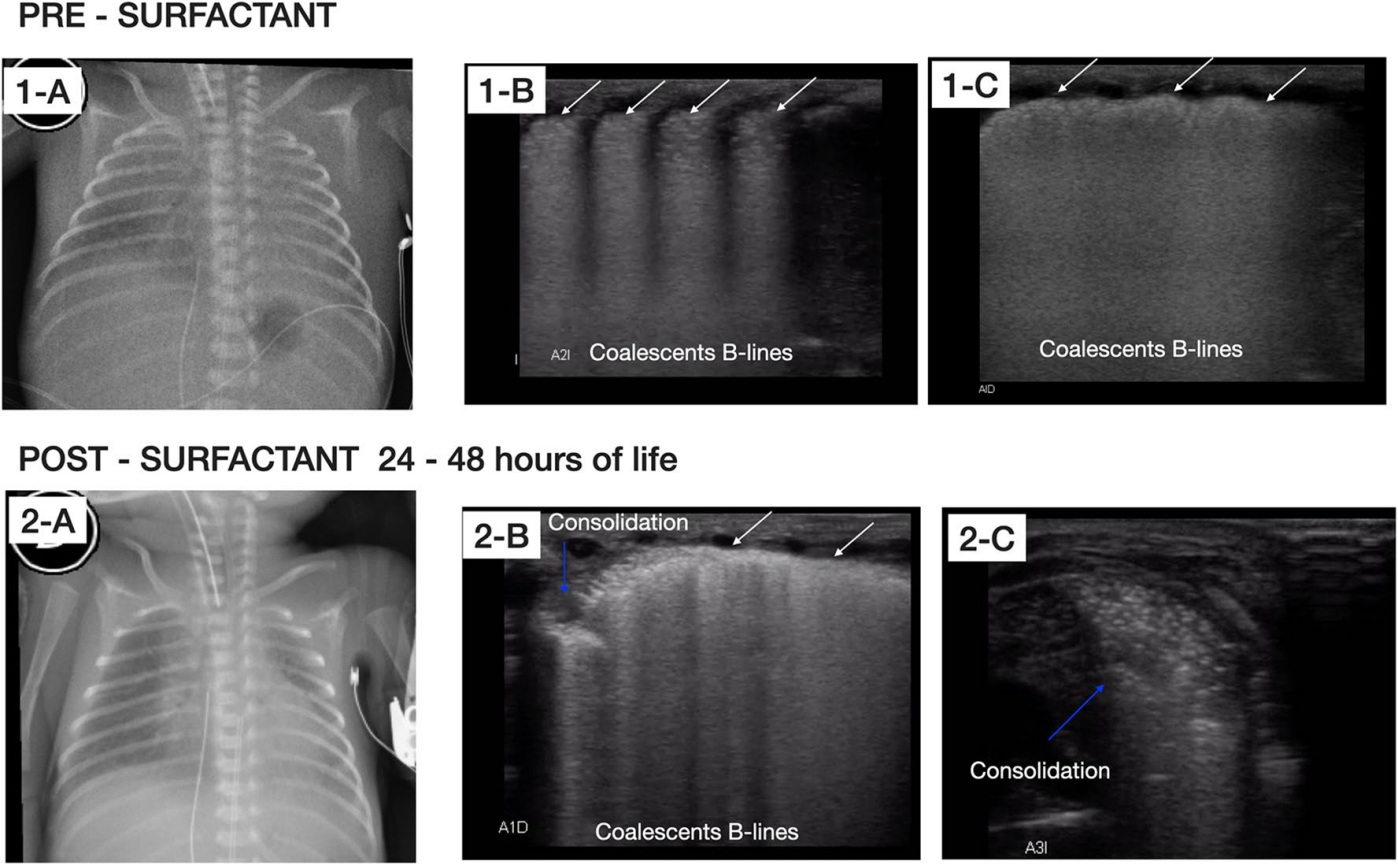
Radiographic and LU examination pre-surfactant administration and after 24–48 h of life. Top: chest X-ray and LU examination after birth pre-surfactant administration. 1-A Chest X-ray shows poorly inflated lungs with ground glass shadowing widespread in both hemithorax with obliteration of cardiac silhouette. 1-B and 1-C LU examinations in longitudinal and oblique view, respectively, show irregularity of the pleural line (white arrows), subpleural consolidation and widespread appearance of “white lung” on both lung fields without spared areas. Bottom: chest X-ray and LU examinations performed after 24–48 h of life. 2-A chest X-ray shows increased interstitial infiltrate. 2-B and 2-C LU examinations in oblique view, show severe deterioration of lung aeration, presence of multiple bilateral consolidations (blue arrows), air bronchograms, and coalescent B-lines without spared areas
